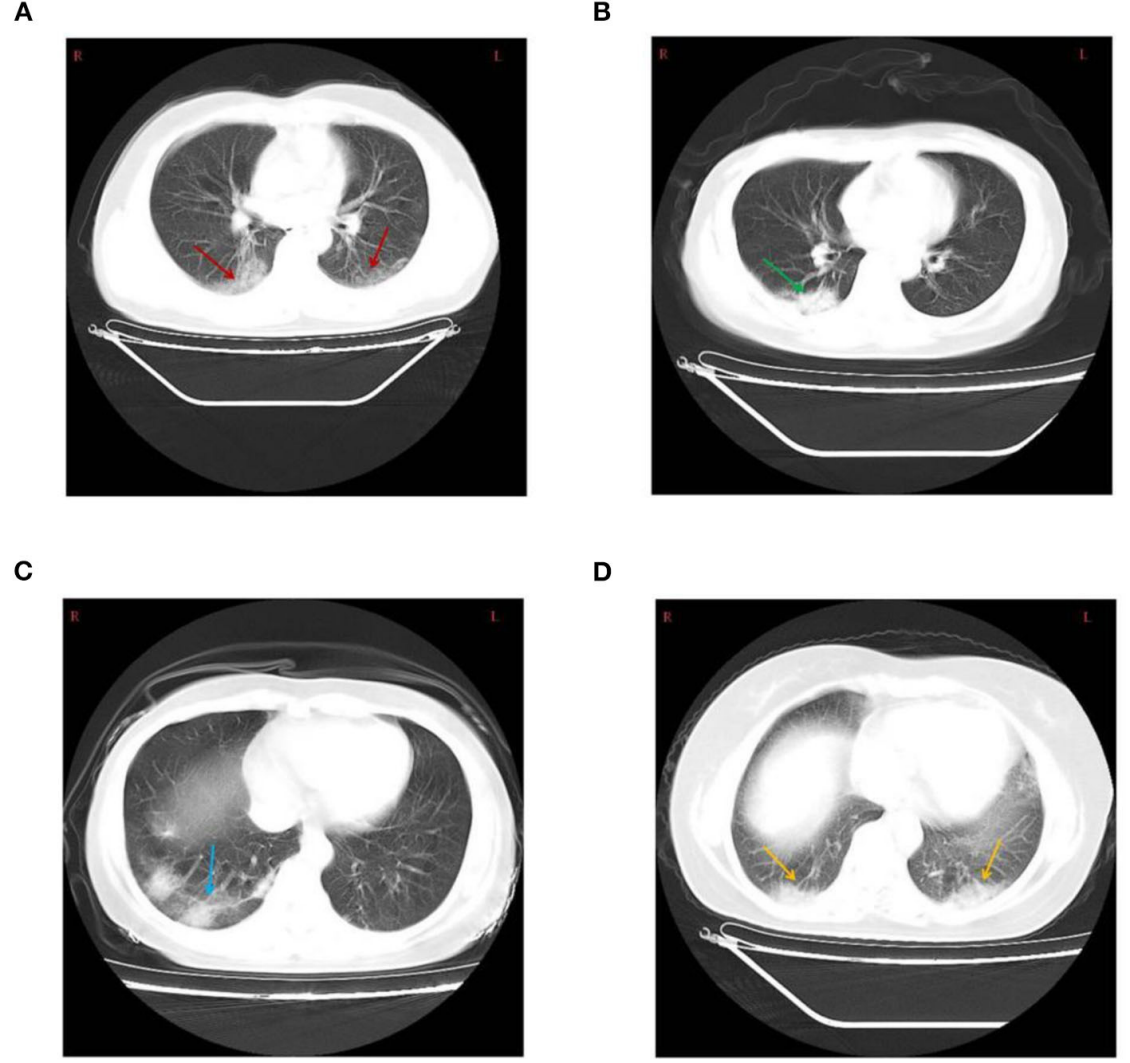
(A) Chest computed tomography showing bilateral diffuse ground-glass opacity (red arrow) in a 43-year-old male patient. (B) Patchy shadow of the right lung (green arrow) in a 44-year-old female patient. (C) Consolidation and Strip-shaped shadows of the right lung (blue arrow) in a 43-year-old female patient. (D) Bilateral lung patchy shadow (yellow arrow) in a 42-year-old female patient.
